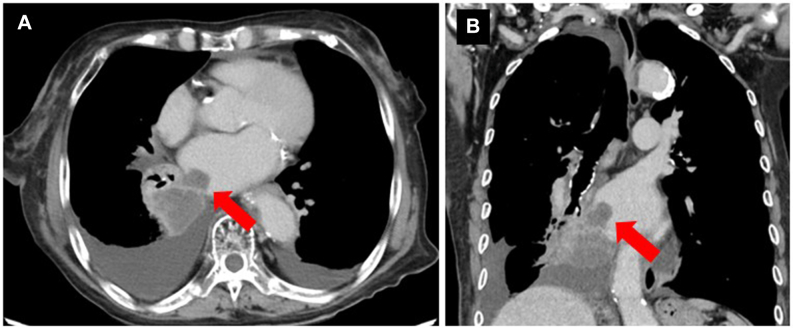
Cardiac CT, axial (A) and oblique coronal (B) views obtained during the portal venous phase, demonstrates the pleural effusions and the large, hypodense SCLC tumor mass with extension from the RLPV into the LA *(arrows)*.
